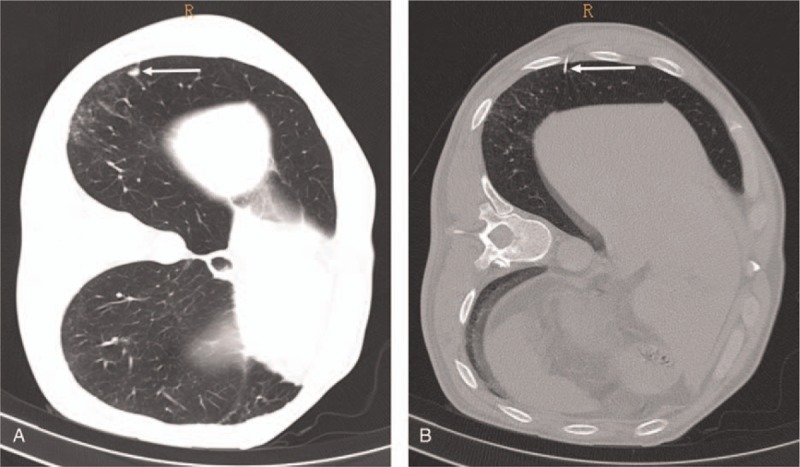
Preoperative computed tomography showing a right pulmonary nodule (A) and intraoperative puncture location (B).
